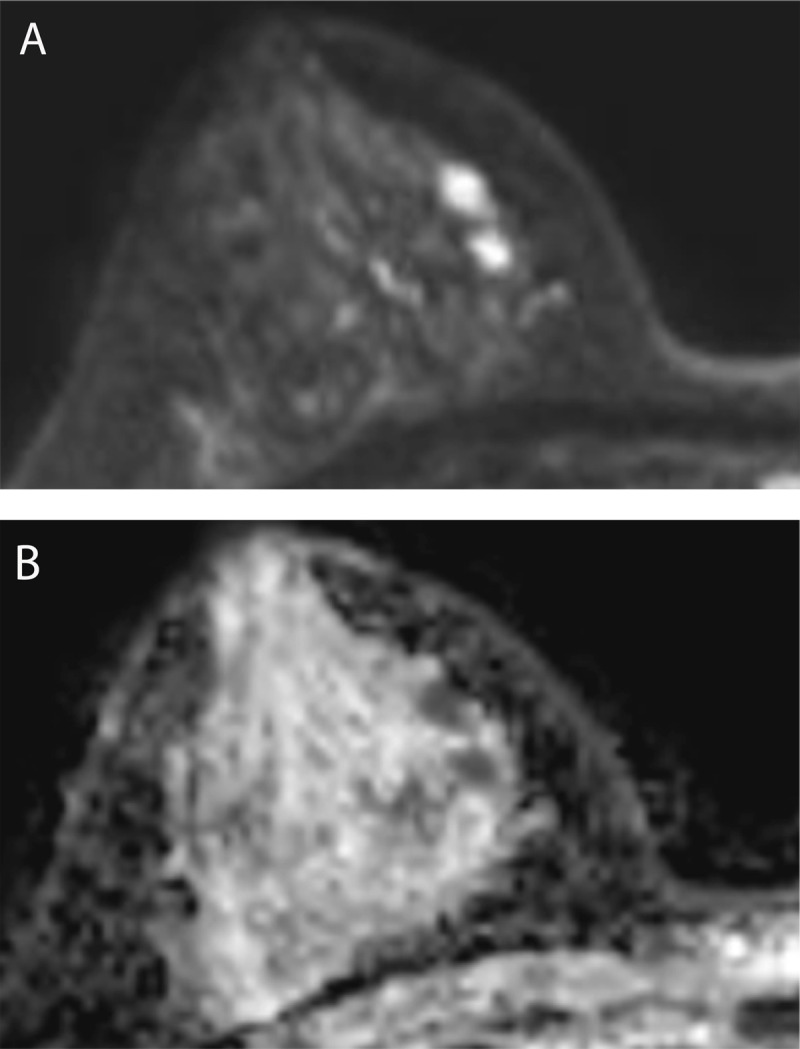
The mass showed a hyperintense signal on DWI with low ADC.
